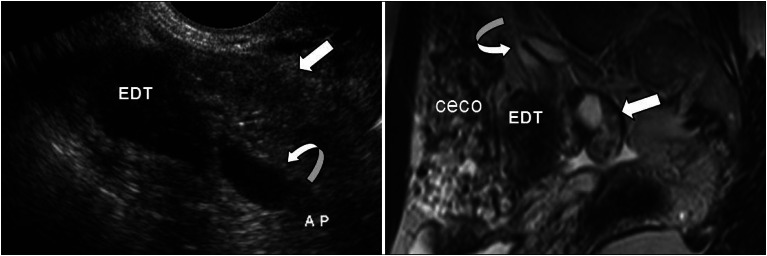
Image of the ileocecal junction and appendix suggestive of endometriosis (EDT), from transvaginal ultrasonography (left) and nuclear magnetic resonance (right). AP = appendix (curved arrow); small bowel shown with the arrow; CECO = ascending colon.
